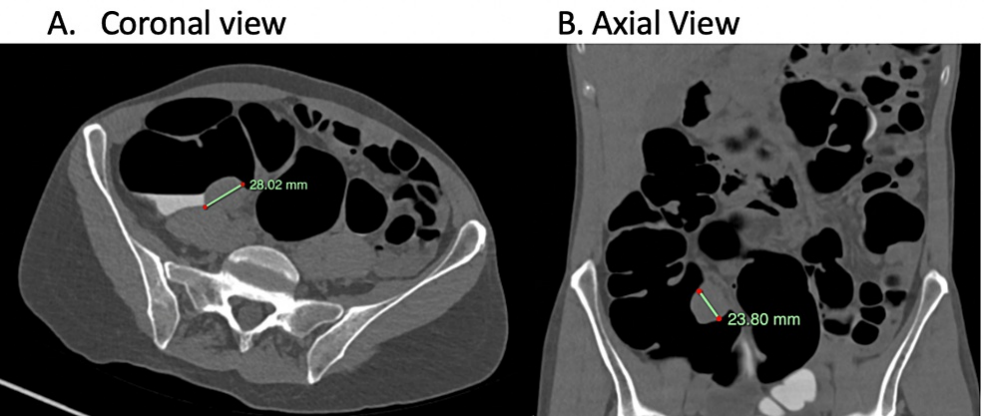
CT colonography. A 28.02 x 23.8 mm circumscribed ovoid soft tissue lesion on the posterior wall of the caecum
